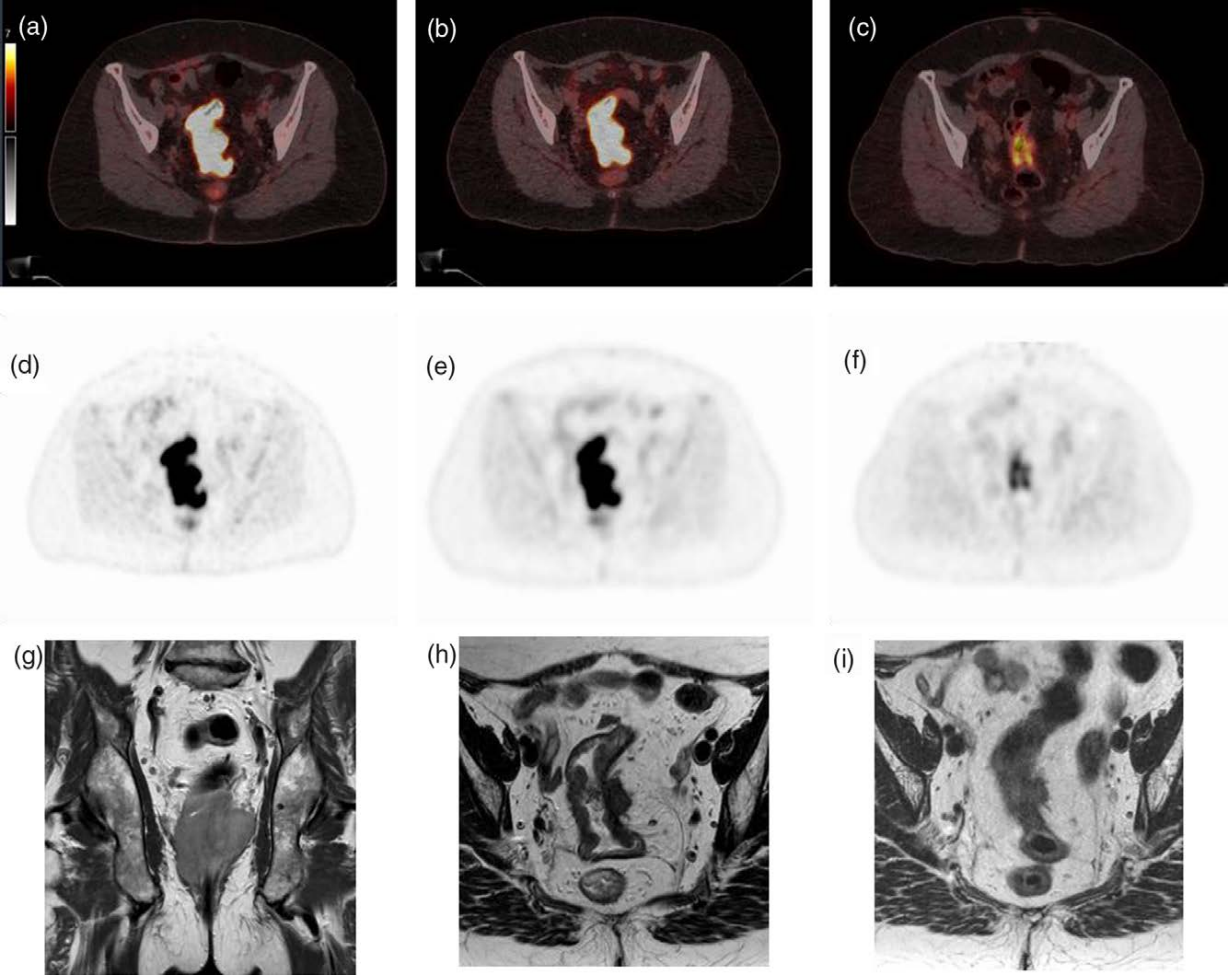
[^18^F]FDG PET/CT and T2 weighted MRI images of a poor responder before, during, and after neoadjuvant chemoradiotherapy. A fifty-eight-year-old woman with cT4aN2M0 rectal cancer had a partial response to chemoradiotherapy to a yiT3N1M0. Pathological examination showed a ypT3N0M0 tumor and pTRG of 4. SUV_max_ was 17.8 at baseline, 17.8 at interim assessment, and 6.5 at reevaluation. Figure shows [^18^F]FDG PET/CT fusion (a–c) and PET-only (d–f) images as well as T2 weighted MRI (g–i) images before (a, d, g), during (b, e, h) and after (c, f, i) neoadjuvant chemoradiotherapy. CT, computed tomography; pTRG, pathological tumor regression grade; SUV_max_, maximum standardized uptake value.
